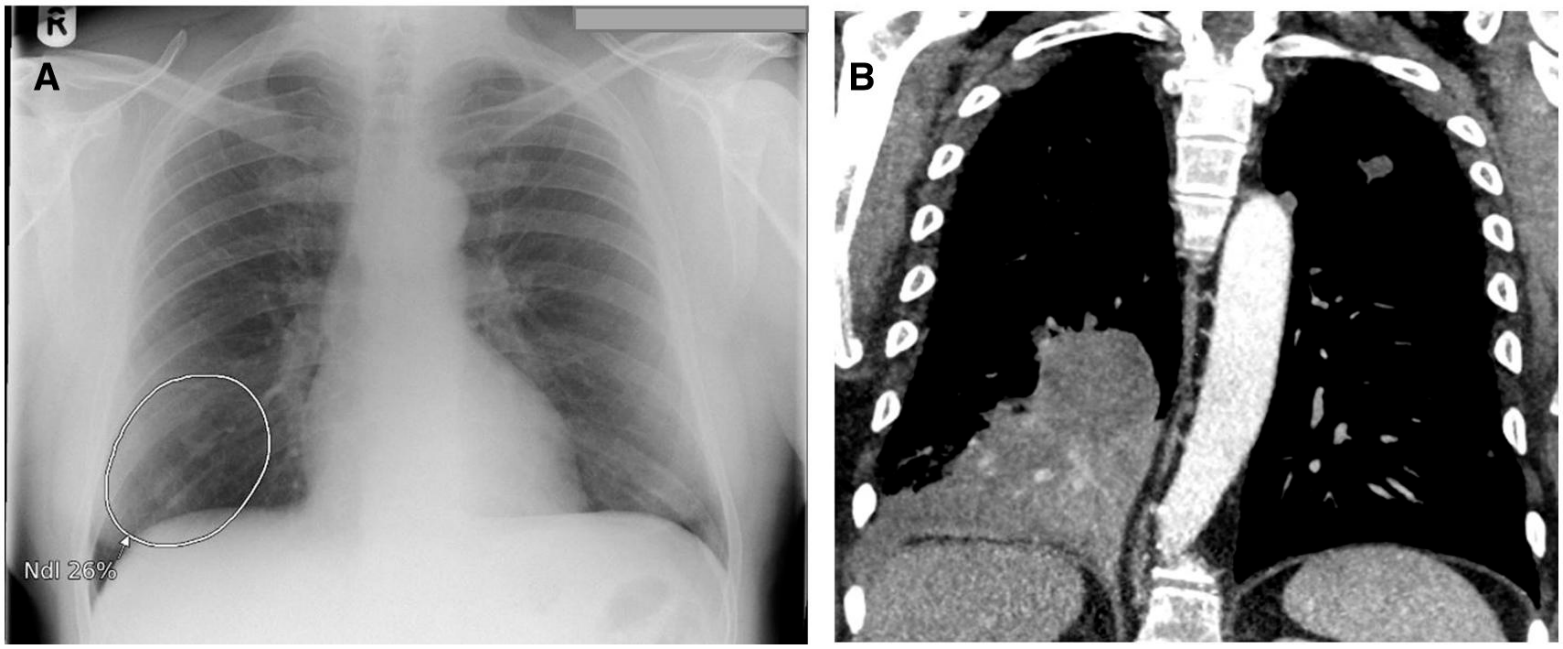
A 62-year-old male with history of smoking and shortness of breath (SOB) underwent a CXR (A), reported as *no abnormility detected (NAD). The AI software highlights suspicion of right lower lobe nodule(s) (white circle) on the same image. Three years and 10 months later, the patient has a CT chest examination (B) following episodes of haemoptysis, demonstrating a right lower lobe mass confirmed to be stage IV LC. (*Note that the NAD code for the purpose of the study denoted no solitary abnormal findings and excluded specific findings of heterogeneous diseases, that is, emphysema, interstitial lung disease). AI = artificial intelligence; CXR = chest radiograph.
